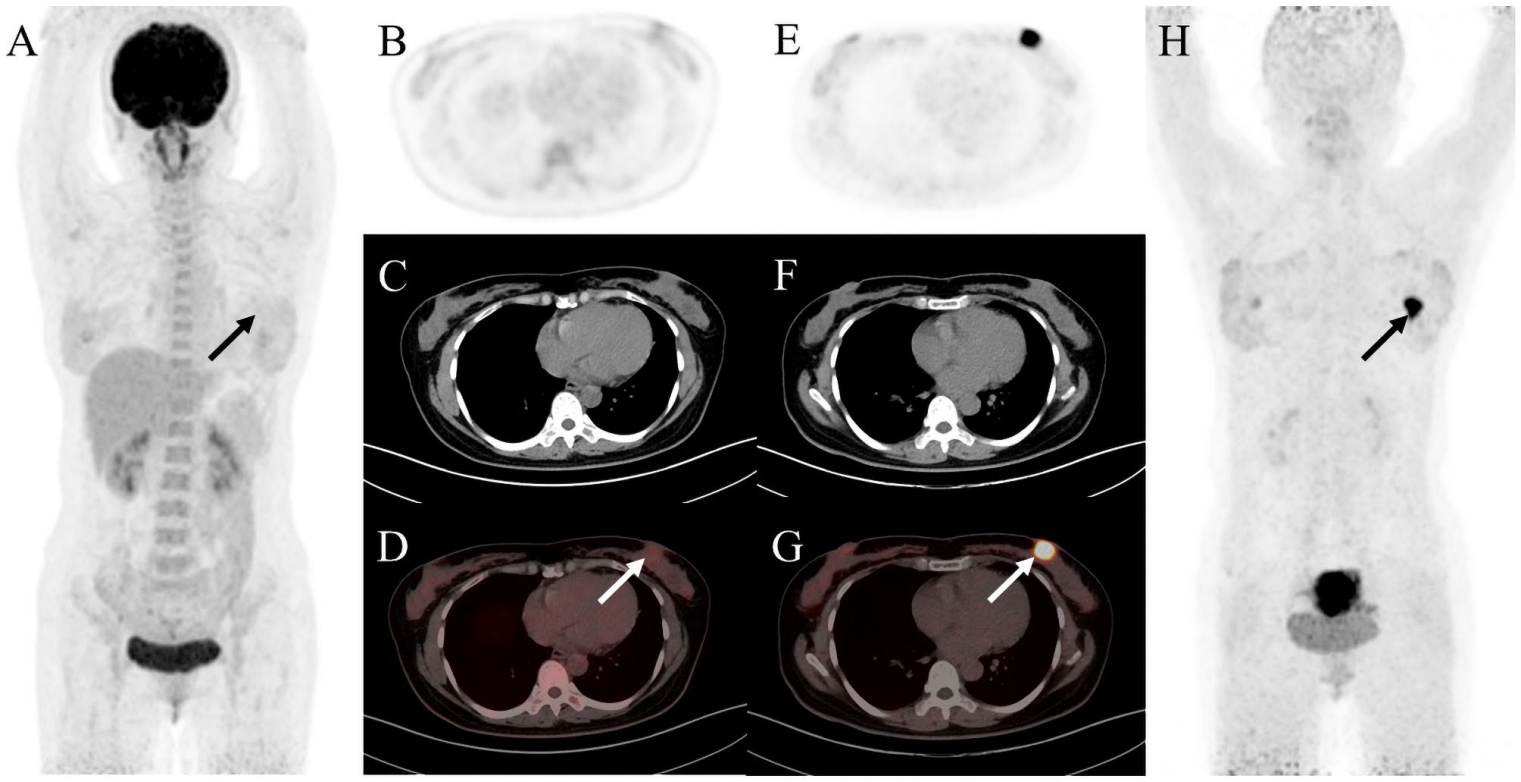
A 47-year-old woman was admitted to our hospital because of left breast mass 20 days ago. For staging, the patient underwent ^18^F-FDG PET/CT. The maximal intensity projection image (A) and the axial views (B: PET image; C: CT scan; D: PET/CT fused image) revealed normal findings. Then she was enrolled in our study and underwent ^68^Ga-FAPI PET/CT. The left breast showed intense uptake on ^68^Ga-FAPI-04 PET/CT (E: PET image; F: CT scan; G: PET/CT fused image; H: MIP, solid arrow; SUV_max_, 15.4). Subsequently, the patient underwent pathology confirmed invasive lobular carcinoma of the left breast
